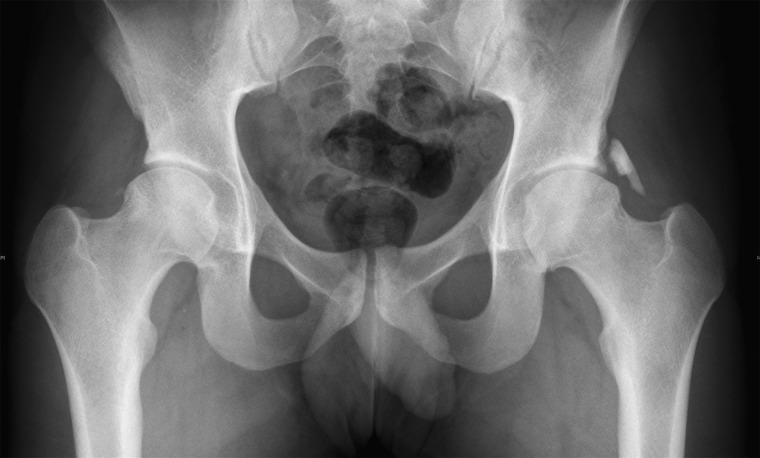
Follow up radiograph of a 20-year-old patient after bilateral hip arthroscopy. The radiograph was taken 9 months status post left and 3 months status post right hip arthroscopy. HO on the left side was evident on radiographs 10 weeks after the index procedure. NSAID prophylaxis using etodolac 600 mg once daily for 2 weeks was administered only after the operation on the right hip.
